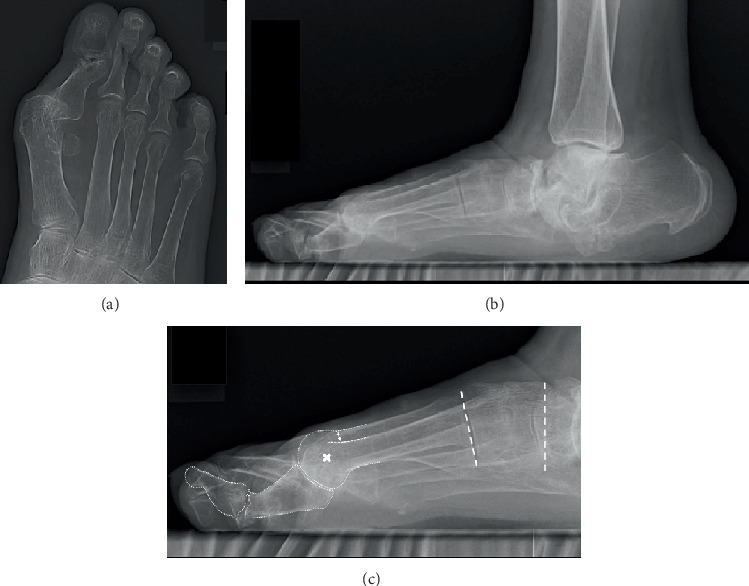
Preoperative weight-bearing radiographs of the foot. (a) Anteroposterior view of the foot showing hallux valgus deformity and no clear space at the metatarsophalangeal joint. (b, c) Lateral view of the foot showing the destruction of the Chopart and subtalar joints, as well as misalignment of the hallux including elevation of the first metatarsal, plantar deviation of the proximal phalanx, and interphalangeal hyperextension. The two-way arrow indicates the distance between two dorsal cortices of the first and second metatarsals, indicating an elevated first metatarsal. The cross indicates the approximate center of the metatarsal head. Dotted lines show the naviculocuneiform and tarsometatarsal joints.
